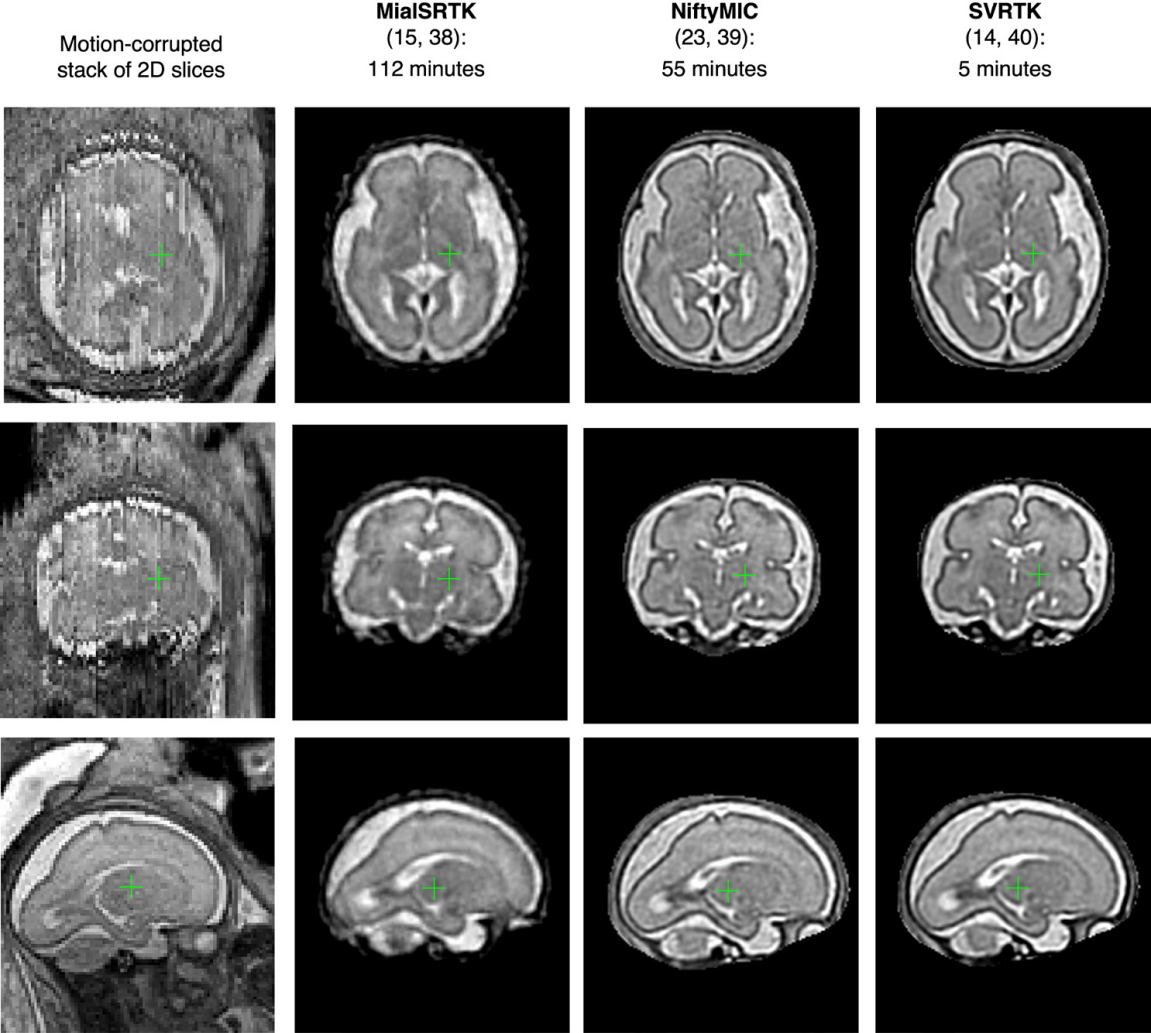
Comparison of different SVR reconstruction toolboxes for foetal brain MRI. SVR, slice-to-volume registration.
